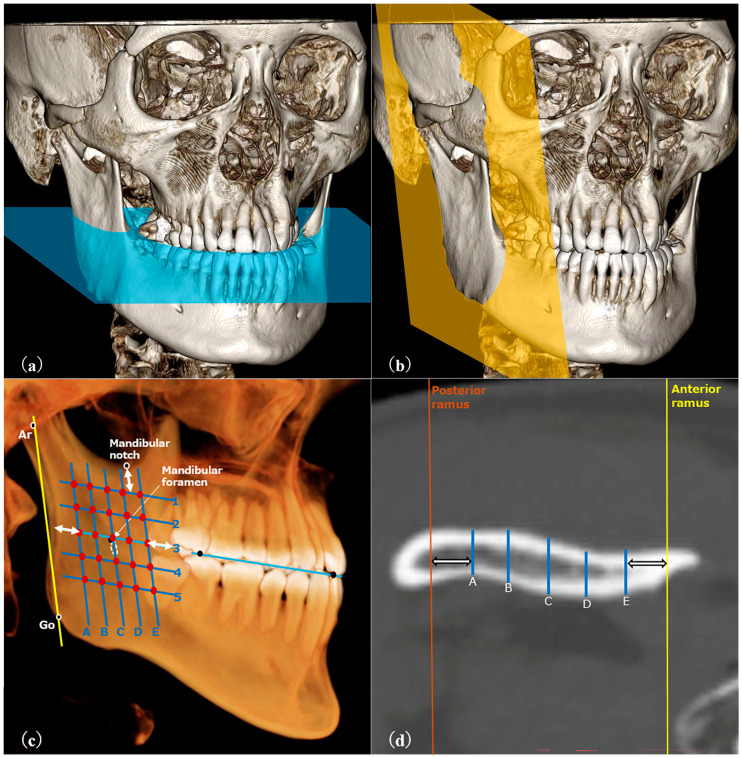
(a–d) CT images viewed with DICOM viewer. (a) The axial plane (blue plane) was parallel to the occlusal plane. (b) The para-sagittal plane (yellow plane) was parallel to the ramus. (c) Five oblique lines, parallel to the ramus’s posterior border and 5 mm from its surfaces, were drawn. Five evenly spaced horizontal lines intersected the middle oblique line 5 mm below the ramus’s top. Thickness was measured at 25 points to average the ramus’s thickness. (d) An example of measurement at a certain cross-section.
